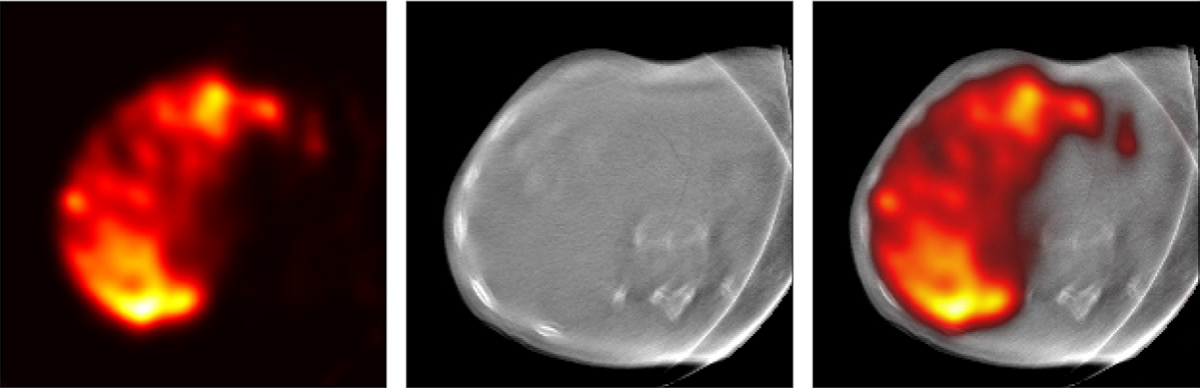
Representative images of a hybrid SPECT (left, right) and cone-beam CT
